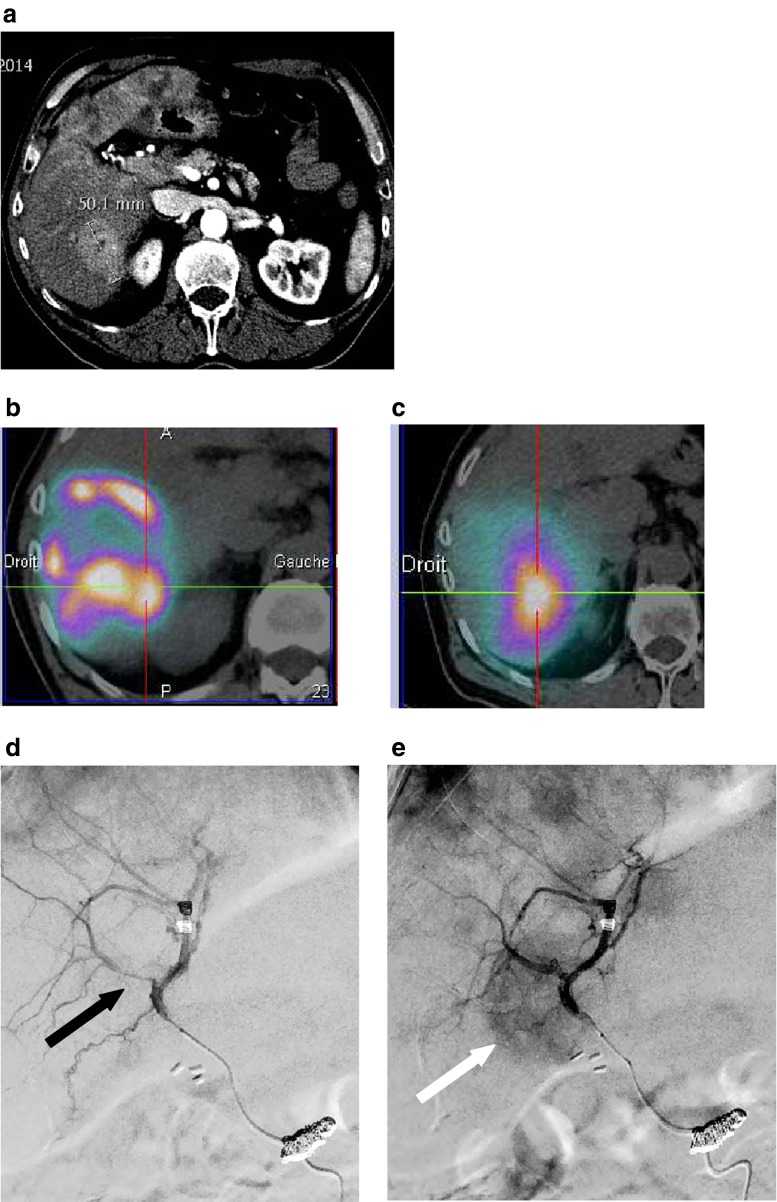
MAA and glass miscrosphere uptake discrepancy related to transient arterial vasospasm. Patient with multifocal neuroendocrine liver metastasis with a 5 cm lesion of segment 6. a) Contrast enhanced CT. b) Tumor MAA SPECT uptake with a “tumor/non tumor” uptake ratio (T/NT ratio) of 0.77 for segment 6 lesion. c) Glass microsphere ^90^Y bremstrahlung SPECT/CT evidencing a better tumor targeting with a T/NT ratio of 3.3 for the same segment 6 lesion. The retrospective analysis of the diagnostic angiography (d) and the therapeutic one (e) shows an arterial vasospasm (*black arrow*) on the diagnostic angiography with a poor tumoral blush as against no vasospasm and a good tumoral blush (*white arrow*) on the therapeutic one
